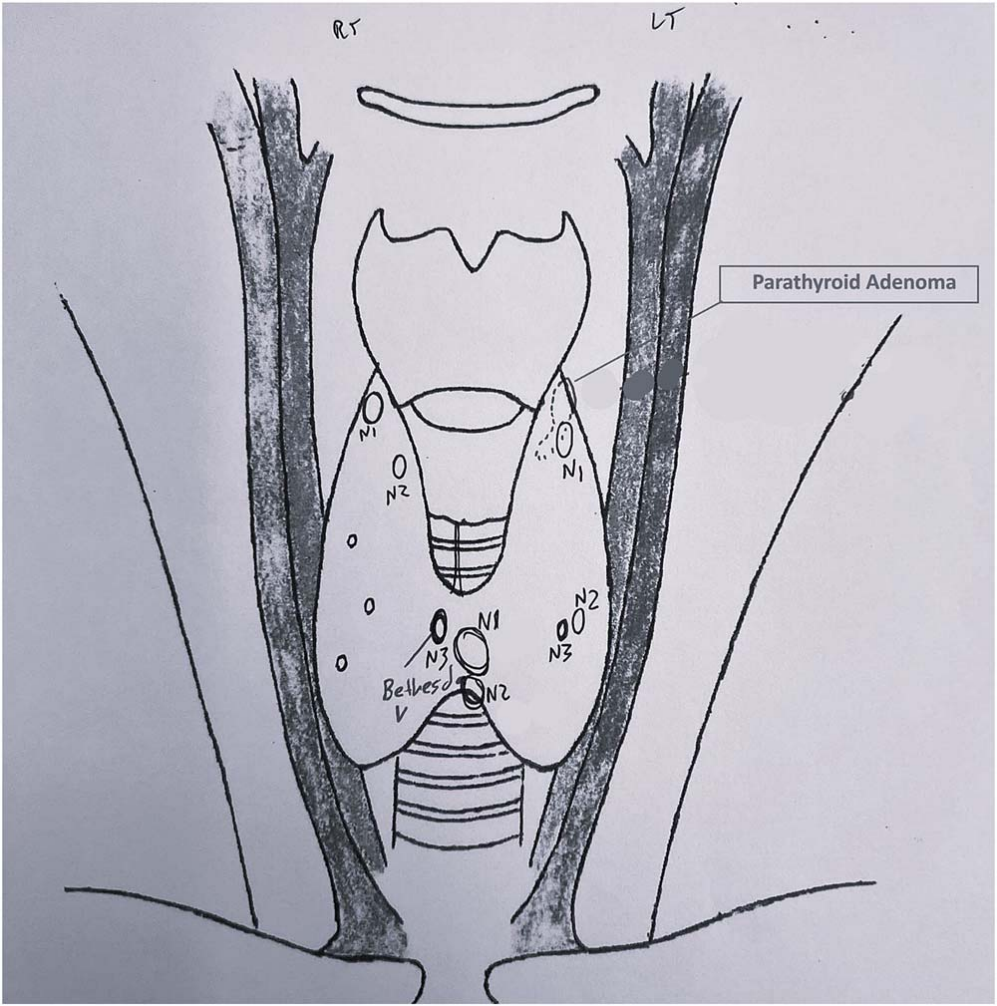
Preoperative ultrasound cervical mapping. Nodular lesions with suspicious sonographic features in both thyroid lobes. The lesions N_1_ and N_3_ in the isthmus were classified as Bethesda III and V, respectively. The parathyroid adenoma is marked behind the left upper lobe.
